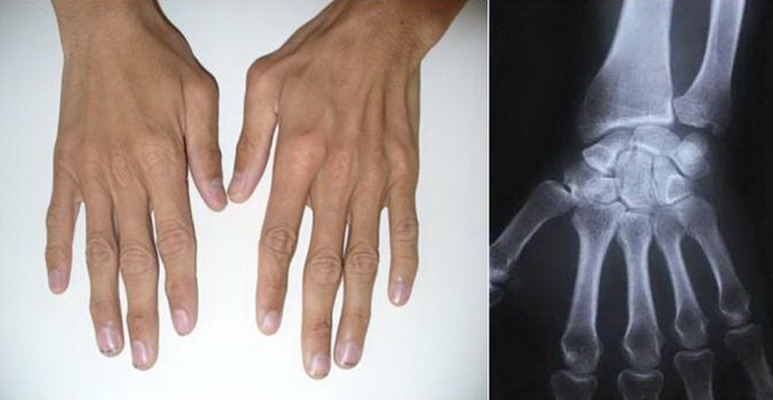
Hand radiograph and photograph showed shortening of the first phalynx of the thumb.
